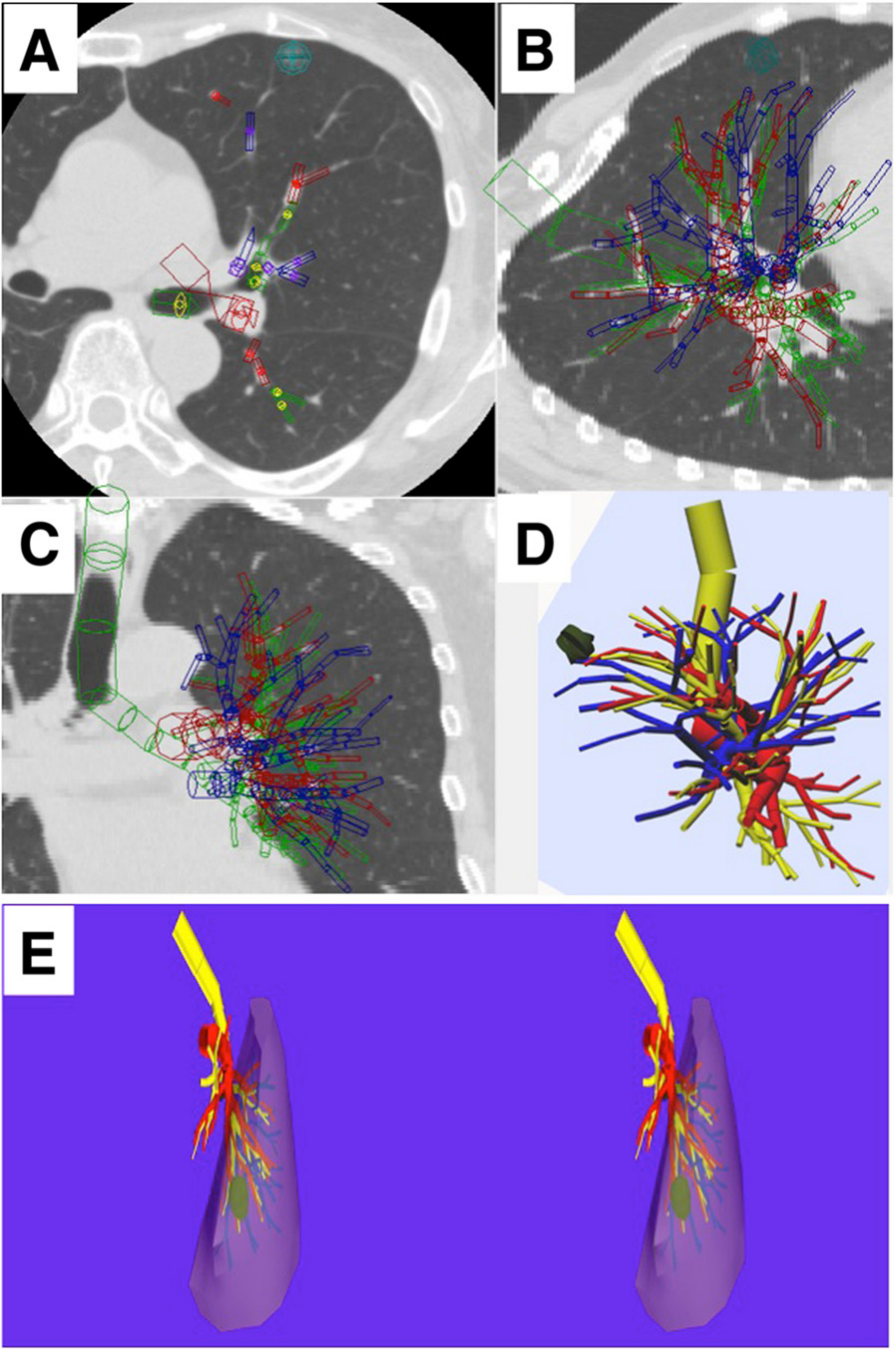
The creation of patient-actual virtual 3-D pulmonary model. (a-c) After the communications-in-medicine (DICOM) format images, which were obtained from 120 of 1-mm thin-sliced high-resolution computed tomography (HRCT)-scan images of tumor and hilum, were uploaded to a personal computer (PC), homemade software “CTTRY” (Tokyo Women’s Medical University, Tokyo, Japan) allowed surgeons to mark pulmonary arteries, veins, bronchi, and tumor on the HRCT image manually and attempt to reconstruct an anatomical model with the help of anatomically correct images. (d) The locations and thicknesses of the pulmonary vessels and bronchi were rendered as various sizes of cylinders. In accordance with the resulting numerical data, a 3-D image was reconstructed with software Metasequoia shareware (http://metaseq.net/). (e) The data of the reconstructed 3-D images was converted with Autodesk® 3ds Max® 2012 (Autodesk, San Rafael, CA, USA). On the PC, reconstructed 3-D pulmonary images were appeared as left- and right- eye view images and also output to a 3-D monitor
